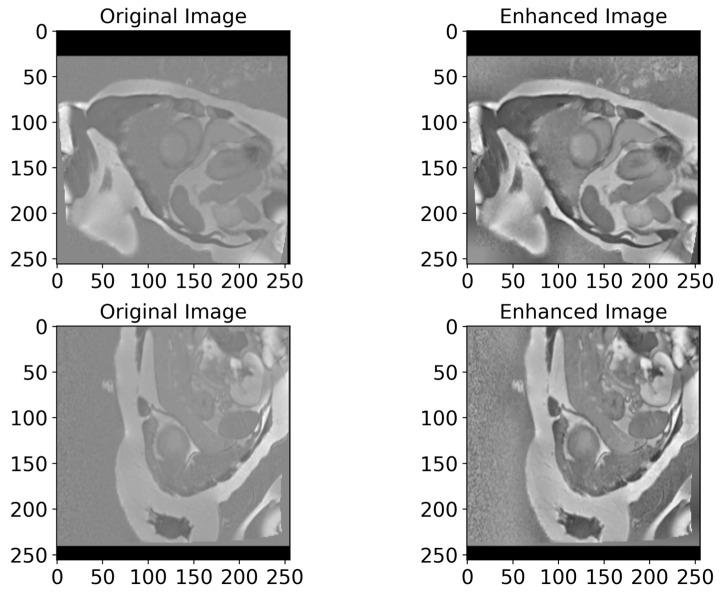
A visualized comparison of original images with enhanced images. The left column presents the original MRI images of the heart, while the right one shows the enhanced images processed utilizing CLAHE and bilateral filtering methods. Visually, the enhanced images demonstrate an increased contrast and clarity, which leads to a more accurate heart segmentation. These visible improvements give DL models the ability to more accurately highlight critical structures and borders of the heart, which in turn improves the diagnosis quality.
